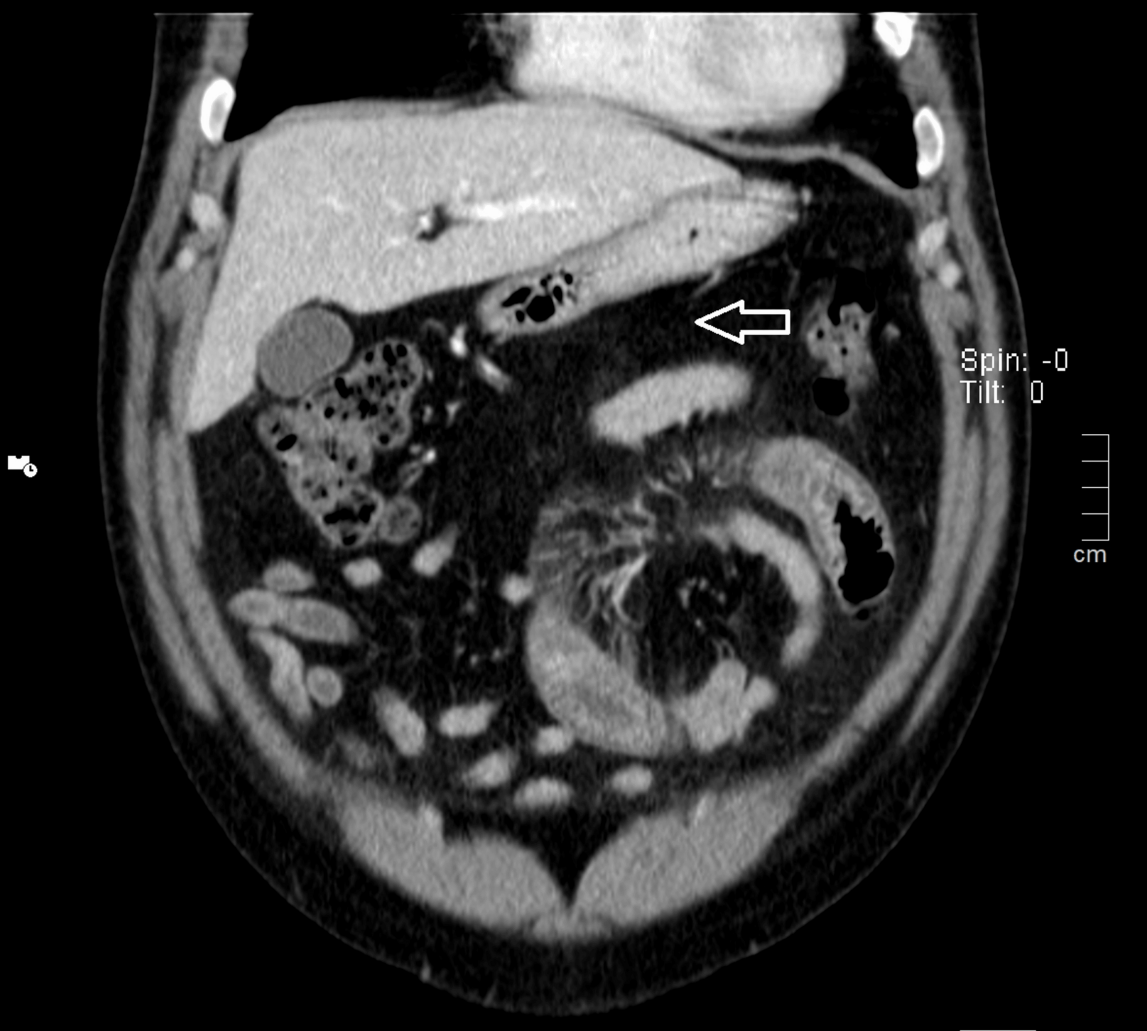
Coronal plane of abdominal CT Circumferential thickening of the jejunal mucosa measuring 5 inches to 9 inches in length is seen associated with densification of the adjacent tissues (arrow).
